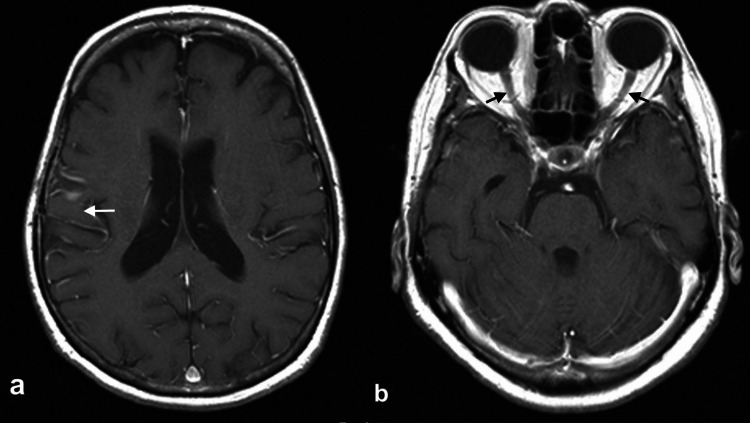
Repeat post-contrast cranial MRI showed (a) no recurrence of intracranial lymphoma (white arrow). (b) Bilateral optic nerves are normal (black arrows).
